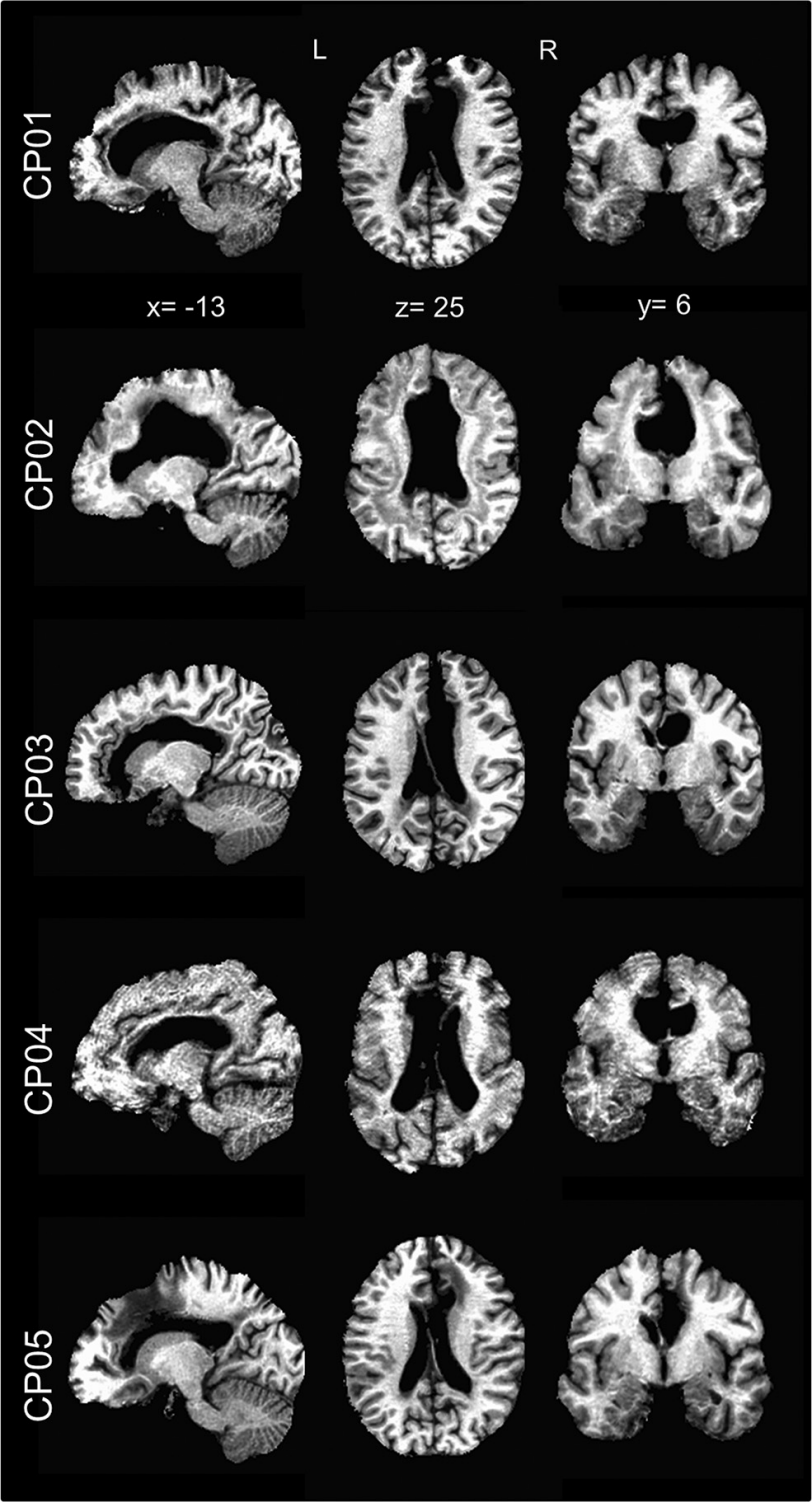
Anatomical MRI images of callosotomized patients. For each patient (CP01–CP05) sagittal, axial, and coronal MRI images are shown in MNI space. It is possible to appreciate the complete absence of the corpus callosum in all cases.
